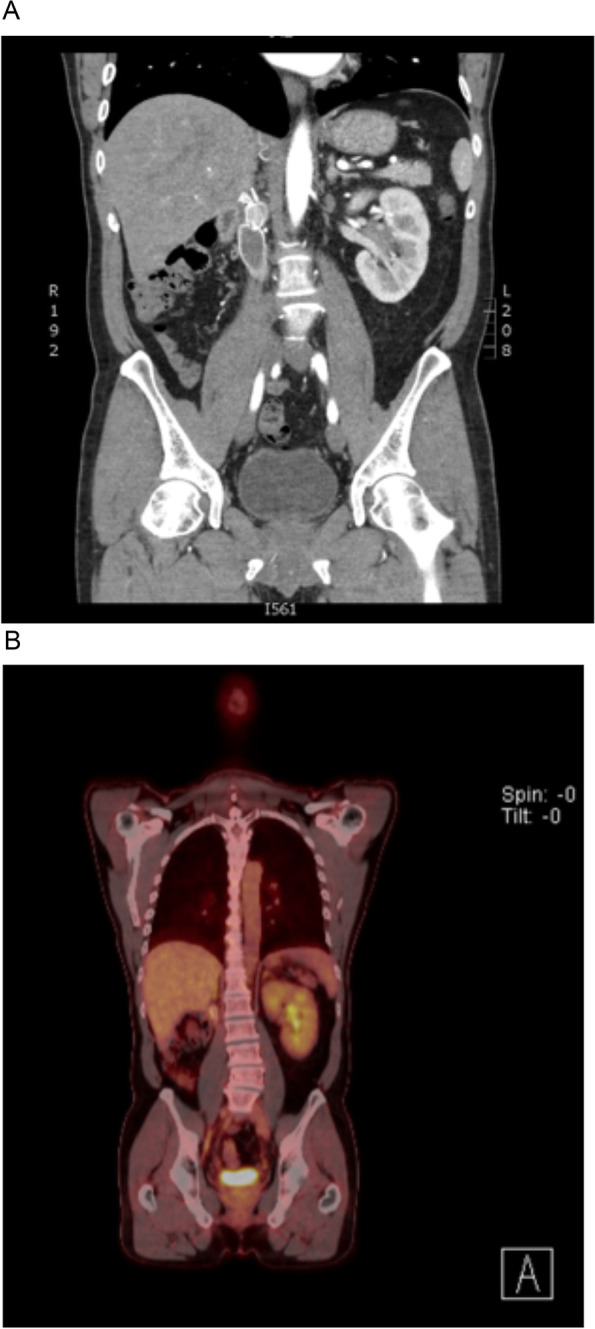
A Post-operative CT. B Post-operative surveillance PET scan. Both demonstrating complete resection of tumour with no residual disease activity
